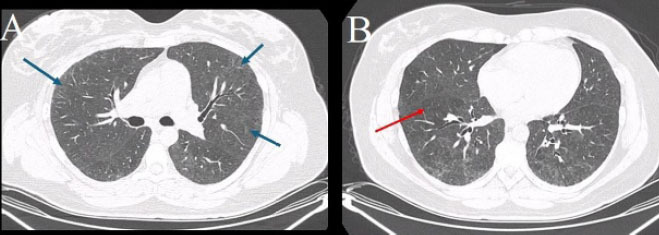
In A, axial chest CT demonstrating bilateral
